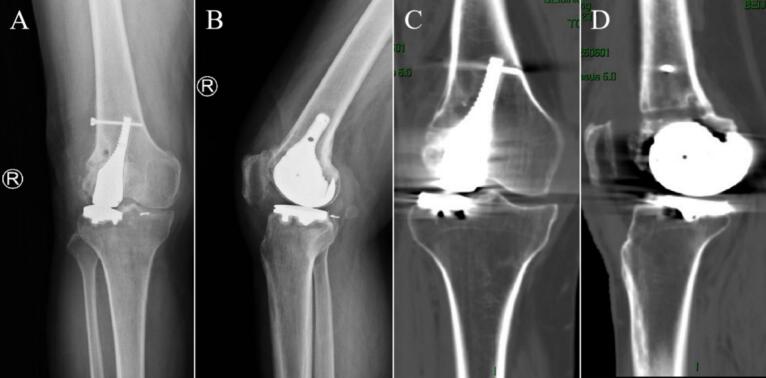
The images at twelfth postoperative weeks. (A) AP view of the right knee X-ray. (B) Lateral X-ray of the right knee. (C) CT AP view of the right knee. (D) CT lateral view of the right knee.
